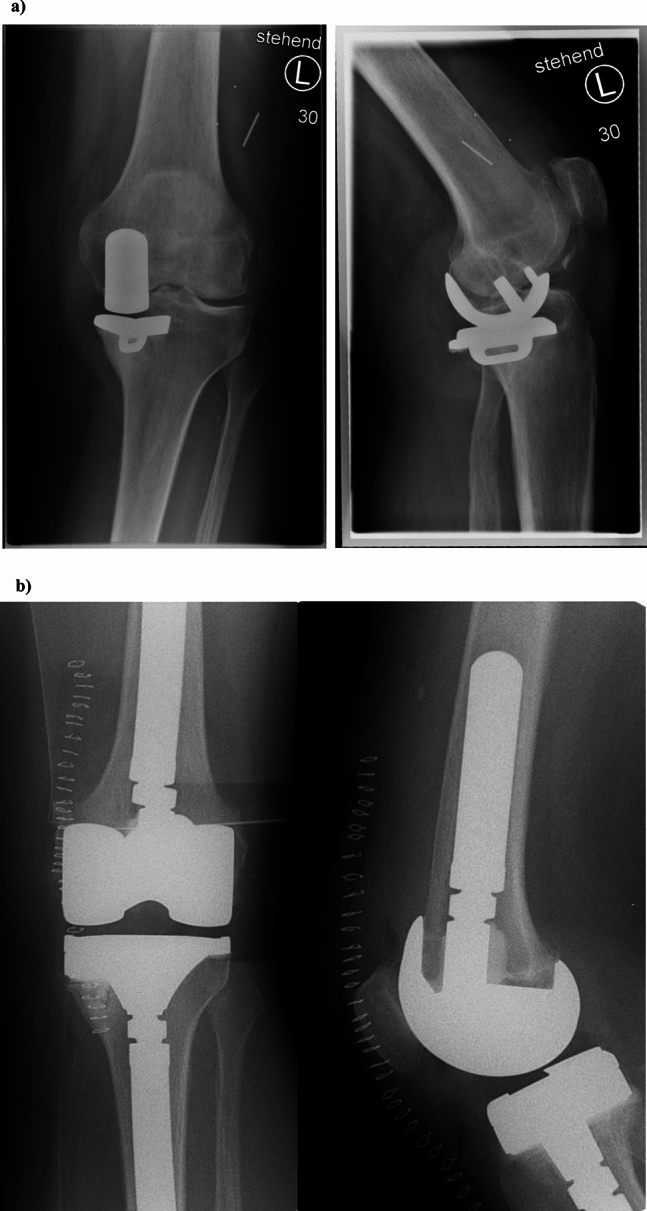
Case 1 shows a 71-year old female patient with a loosening of a medial hemiprosthesis with a consecutive bony KDC 2 defect of the tibia and medial instability KDC B. a Preoperative x-rays anterior–posterior view on the left and lateral view on the right. A mal rotated hemiprosthesis with loosening can be seen. Clinically the patient also displays a medial instability with a high likelihood for bony as well as ligamentary damage (KDC B). b Postoperative x-rays anterior–posterior view on the left and lateral view on the right. The tibial KDC 2 bone defect has been treated with a block augment (10 mm) creating sufficient force transmission and rotational stability in addition to a modular un-cemented stem. A semi-constrained implant was chose due to remaining instability after anatomic reconstruction of the joint line
